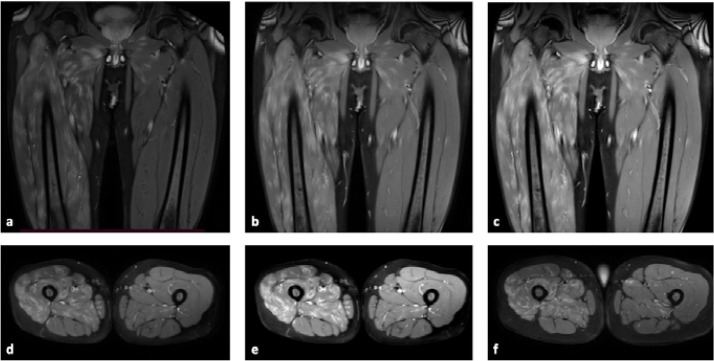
Coronal (a) proton density weighted (PDW) with fat saturation, (b) contrast enhanced T1-weighted fat saturation and (c) T1-weighted; and axial (d) proton density weighted (PDW), (e) contrast enhanced T1-weighted fat saturation and (f) T1-weighted MRI of the thigh muscles at presentation. There is remarkably increased intensity and swelling within the muscles representing severe oedema and inflammation more prominent on the right side.
